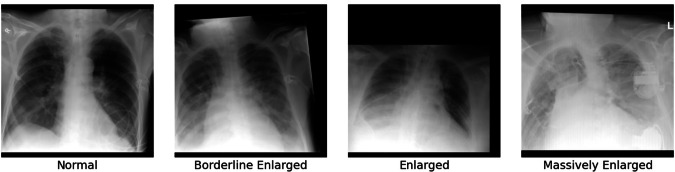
Example Radiographs with Different Labels for “Heart Size”. Heart size was graded as normal, borderline enlarged, enlarged, or massively enlarged. In clinical contexts, enlarged heart size is usually referred to as “cardiomegaly”.PulmonaryCongestion: Severity grading for pulmonary congestion, encoded as per Table 1. Examples of the different grades are given in Fig. 2.
